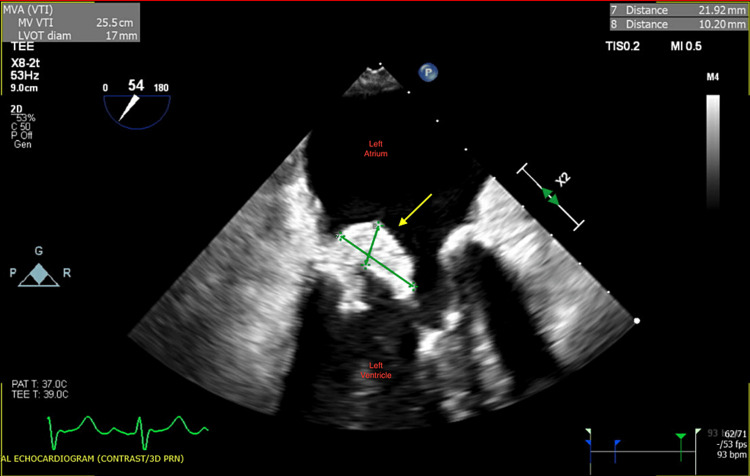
Transesophageal echocardiogram showing enlargement of the vegetation (2.1 cm) on the anterior leaflet of the mitral valve
